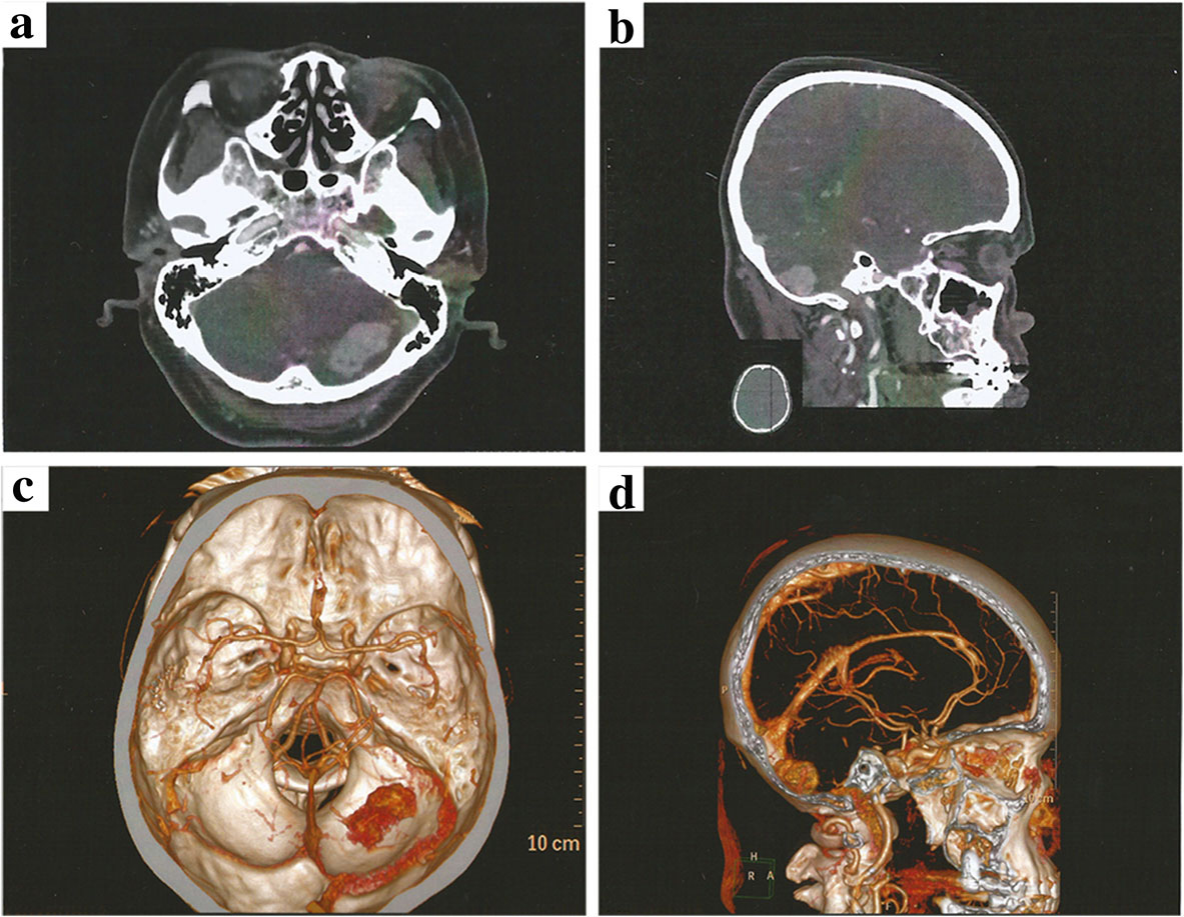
Imaging examination results. Contrast-enhanced CT reveals an oval mass with heterogeneous enhancement in the right cerebellum. (a: coronal view; b: sagittal view). CTA showed tumor with abundant blood supply (c: coronal view; d: sagittal view)
